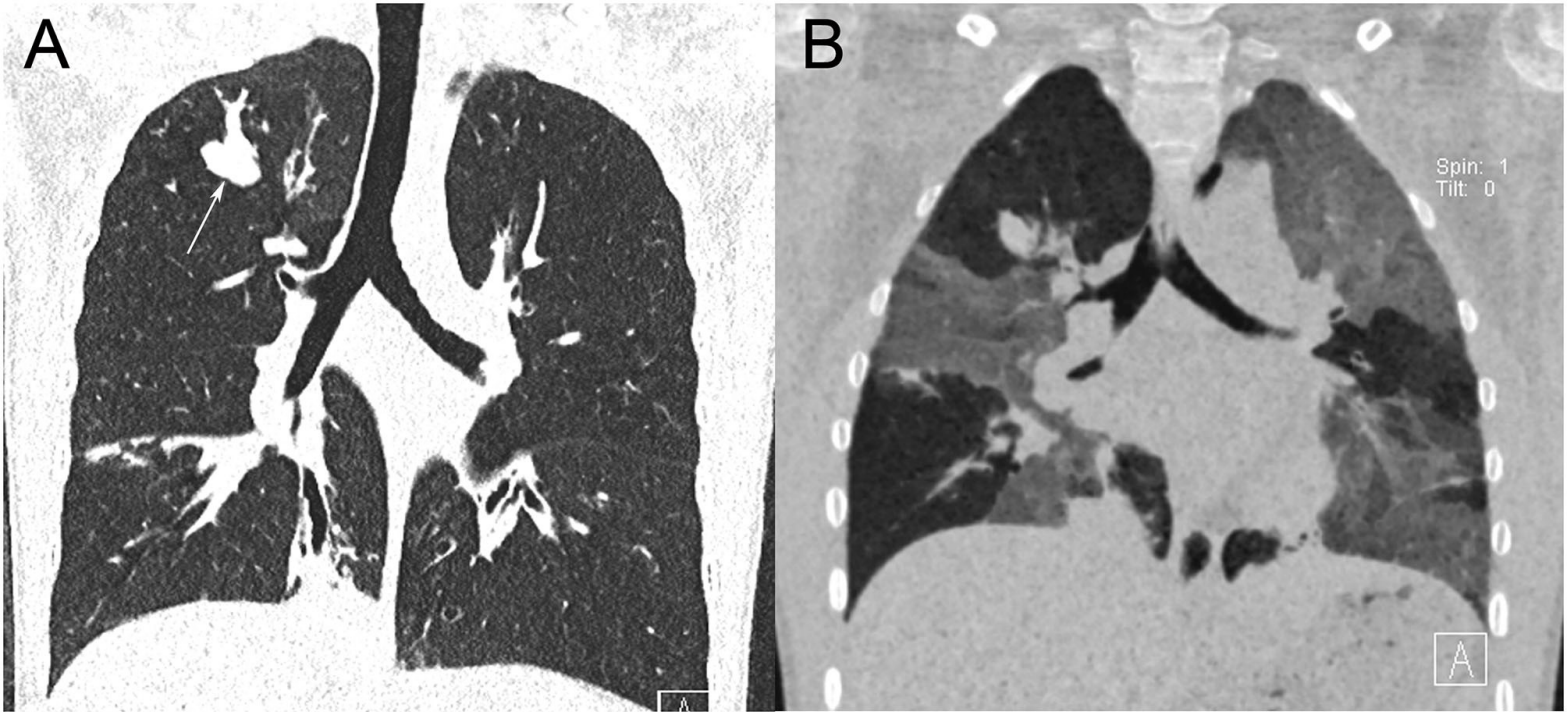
Chest CT in a 13-year-old female with cystic fibrosis. (A) Coronal inspiratory CT image shows upper and lower lobe bronchiectasis. A bronchus in the right upper lobe and its branches are markedly distended with a mucus plug (arrow). (B) Coronal expiratory minimum intensity projection (MinIP) image shows widespread but patchy air trapping, indicating small airways disease.
